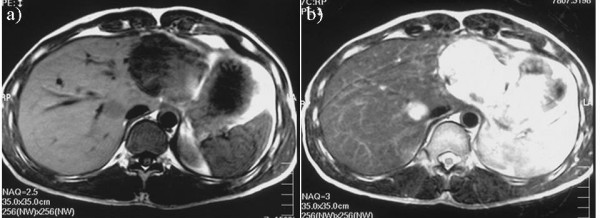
Abdominal MRI in 2004, showing the unilocular cyst 10 cm in diameter. a) low intensity, partly high intensity on T1-weighted image, b) high intensity on T2-weighted image were seen.
